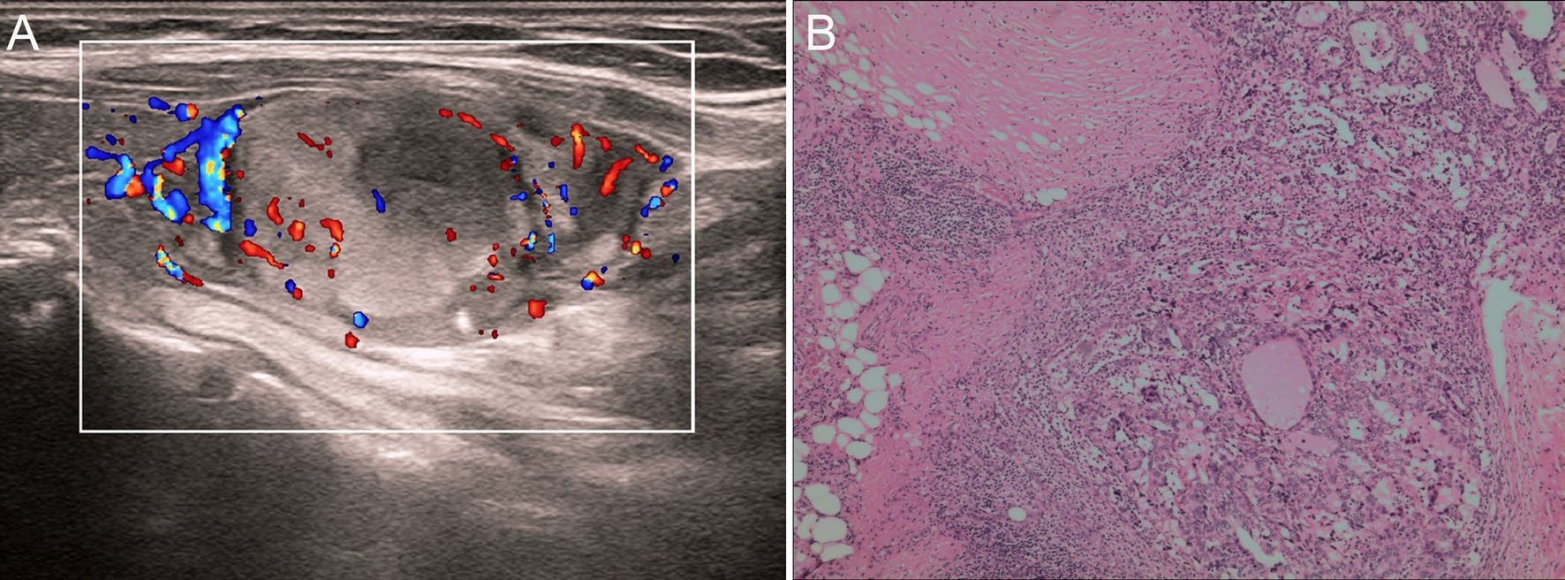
Ultrasound and pathological examination of thyroid tumors. A An ultrasound examination revealed a hypoechoic nodule, measuring 36 × 17 x 21 mm, situated in the left lower pole of the thyroid gland. It exhibited well-defined borders and regular structure. The nodule was filled with intense blood flow signals. B The pathological examination indicated a microinvasive follicular carcinoma of the thyroid. Tumor cells penetrated the thick fibrous capsule. (Amplification: × 40)
